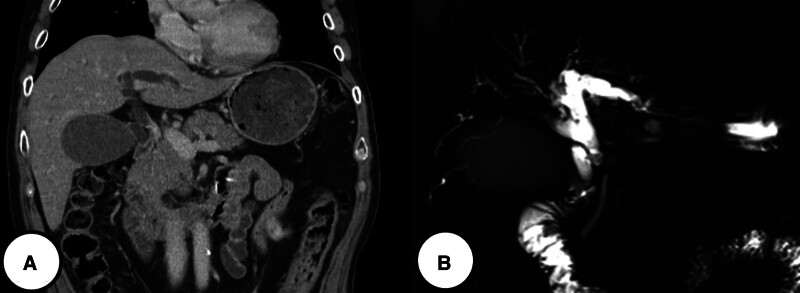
Abdominal image scan of the second case patient showing biliary obstruction just below cystic duct confluence including 2 cm length mass. (A) Abdominal computed tomography scan. (B) Magnetic resonance cholangiopancreatography scan.
